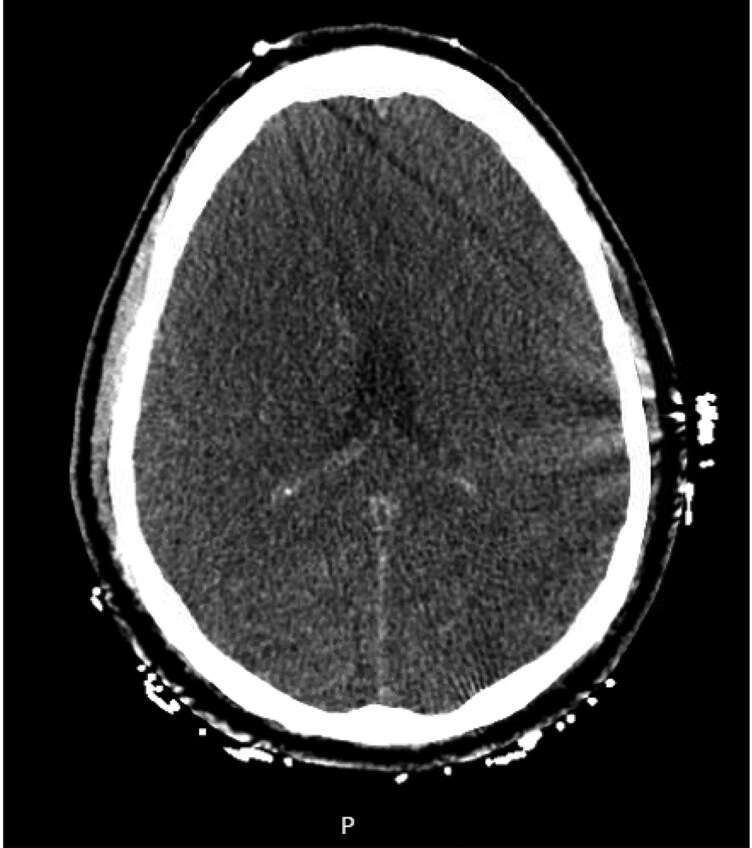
An image from the patient’s CT head showing loss of grey–white matter differentiation and sulcal effacement, consistent with global anoxic brain injury.
